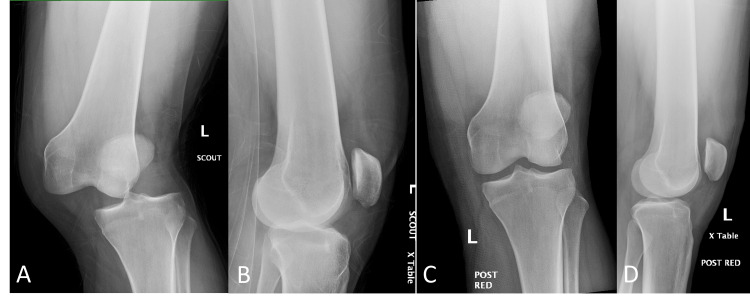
Lateral displacement of the left tibia and fibula in relation to the femur in anteroposterior (A) and lateral (B) radiographic views. Post-reduction images reveal anatomical alignment (C, D).
